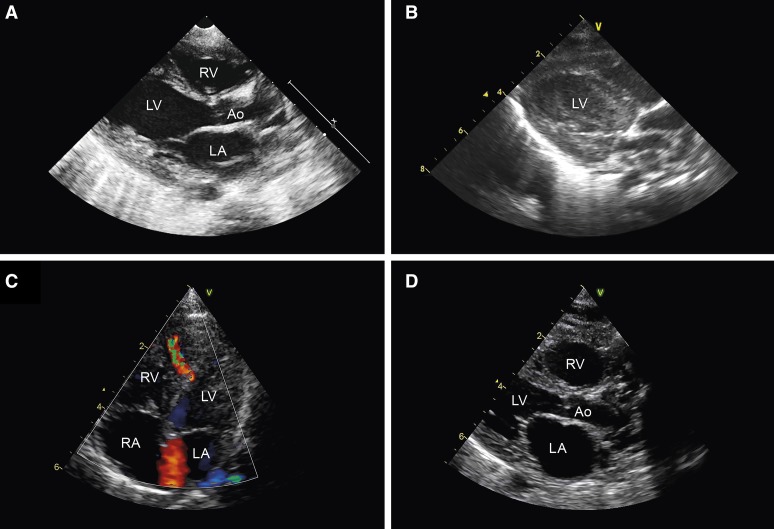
Cardiac ultrasound examination. Patient II:2 (A) parasternal long-axis view during extracorporeal membrane oxygenation (ECMO) showing mild dilatation of the left ventricle; (B) intracardiac thrombus formation. Note: images from the echocardiogram made before ECMO were not available. Patient II:3 (C) 4-chamber view at first day postpartum showing a midmuscular ventricular septal defect. D, Parasternal long-axis view during cardiopulmonary resuscitation showing dilatation of the heart chambers. Ao indicates aorta; LA, left atrium; LV, left ventricle; RA, right atrium; and RV, right ventricle.
